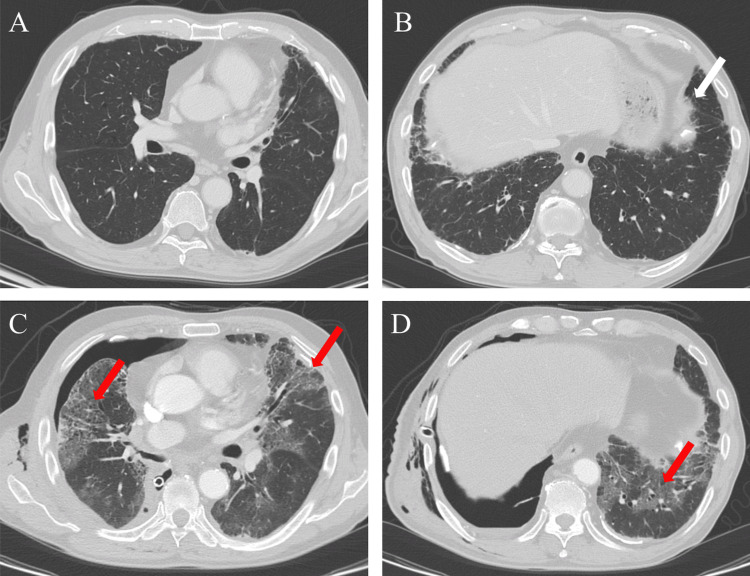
Perioperative high-resolution computed tomography images. (A, B) Preoperative computed tomography image demonstrating indeterminate for usual interstitial pneumonia pattern. White arrows showing lower-lobe subtle reticular abnormality without honeycombing in the subpleural areas with a basal predominance. (C, D) Chest computed tomography scan taken four days after lung surgery. Red arrows showing widespread diffuse bilateral ground-glass opacity with bronchial dilatation.
